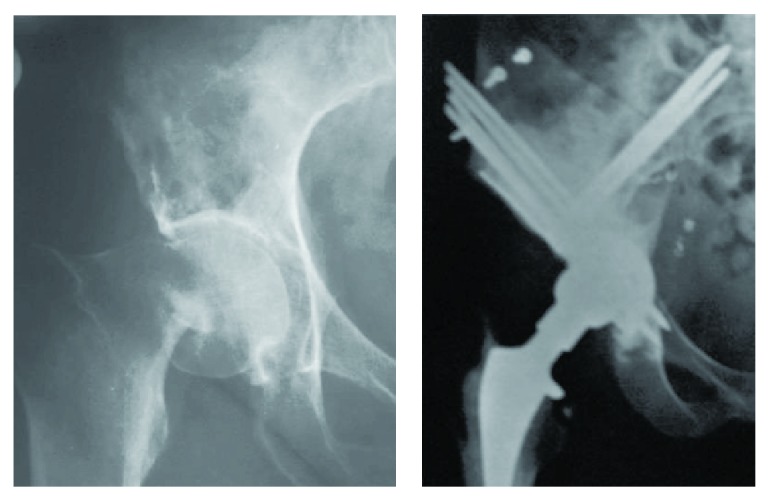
Pre- and postoperative radiographies of Class 3 acetabular defect using the Harrington technique for reconstruction.
